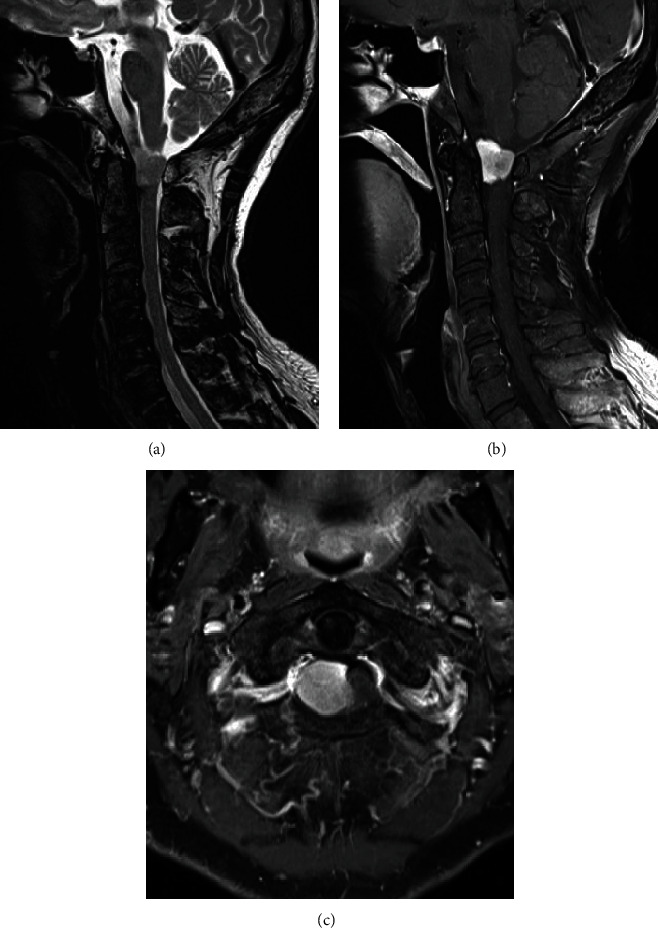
(a) T2-weighted sagittal MRI of the cervical spine showed a tumor in the craniocervical junction. (b, c) MRI followed by the intravenous administration of gadolinium-diethylenetriaminepentaacetic acid (Gd-DTPA) showed a homogeneously enhanced intradural tumor without obvious dural tail sign.
